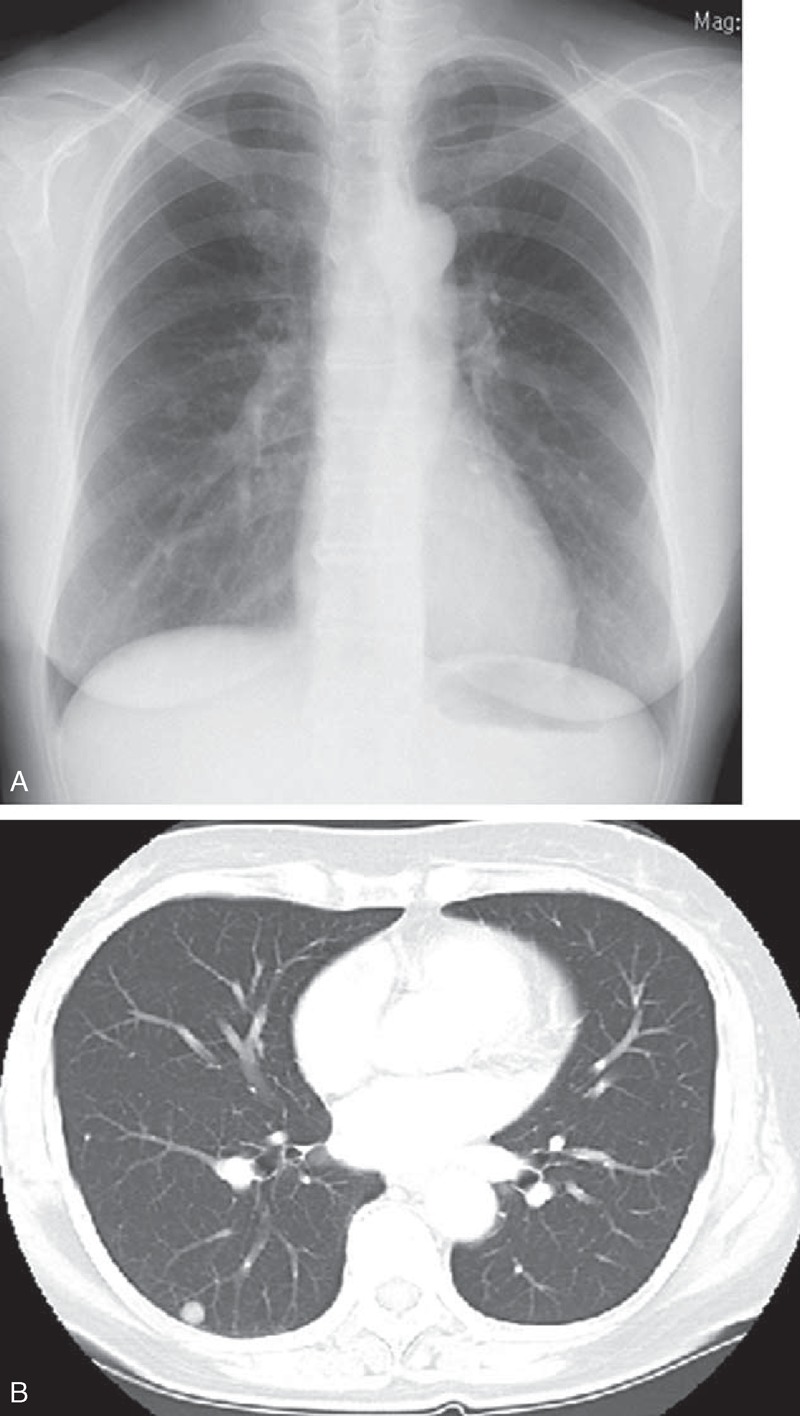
(A) Chest radiograph upon admission to our hospital showing a well-defined nodular shadow in the right middle lung field. (B) Chest computed tomography scan showing a well-defined, round, solid nodule of 10 mm size, located in the right segment 6.
